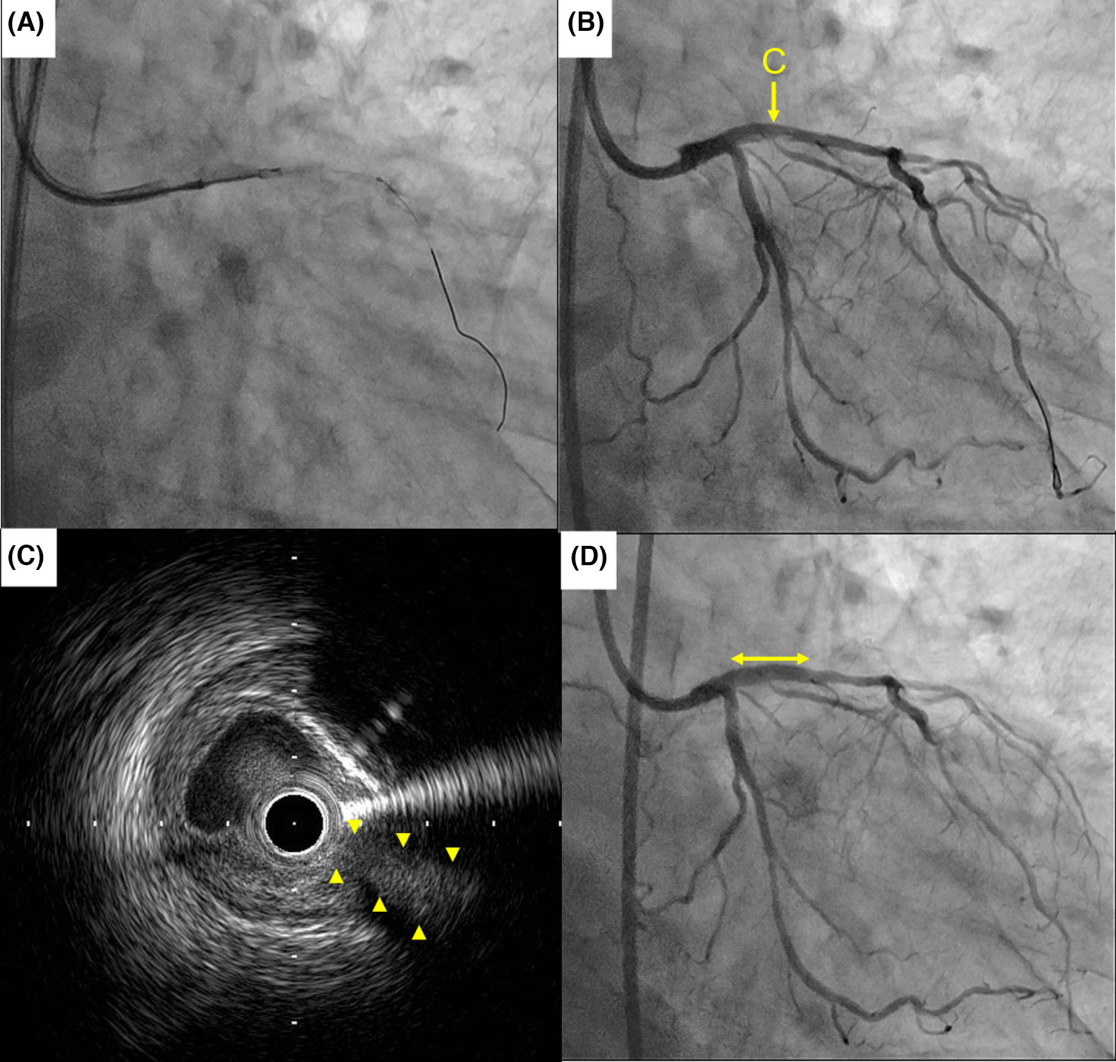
Fluoroscopic and IVUS images following atherectomy. (A) The DCA catheter; (B) Coronary angiography following atherectomy. Arrow C indicates the location of the IVUS images; (C) A cross‐sectional image of the ostium of the side branch (arrowheads); (D) The final coronary angiography. The bidirectional arrow indicates the position of the stent implantation. IVUS = intravascular ultrasound; DCA = directional coronary atherectomy.
